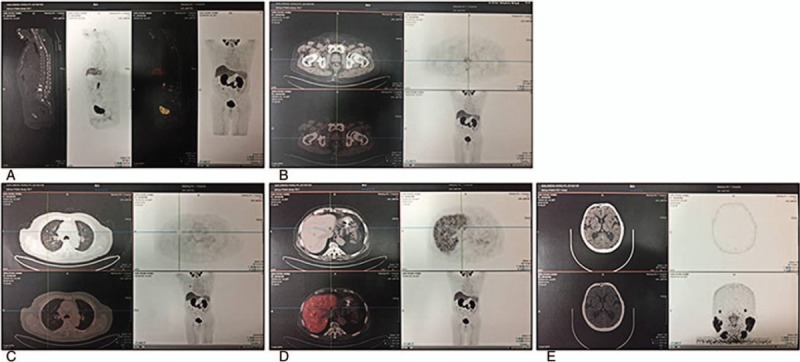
PET-CT scans reflect body (A), prostate (B), lung (C), liver (D), and brain (E) cancer-free metastasis.
